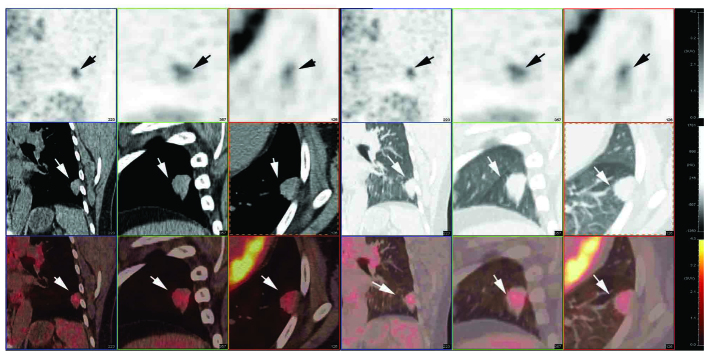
Primary pulmonary amyloidosis on 18F-FDG PET/CT. The PET/CT images revealed a 1.83×1.40 cm lobulated nodule with burr-like margins in the left lower lung exhibiting increased FDG uptake. Continuous slice images are shown from left to right (CT and PET/CT fusion images of the mediastinal window and lung window are shown, respectively). Upper row, PET images (arrows indicate lesions with increased FDG uptake); middle row, CT images (arrows indicate lobulated nodules with burr-like margins); lower row, PET/CT fusion images (arrows indicate lesions with increased FDG uptake in the lobulated nodule). FDG, fluoro-deoxyglucose; PET/CT, positron emission tomography/computed tomography.
